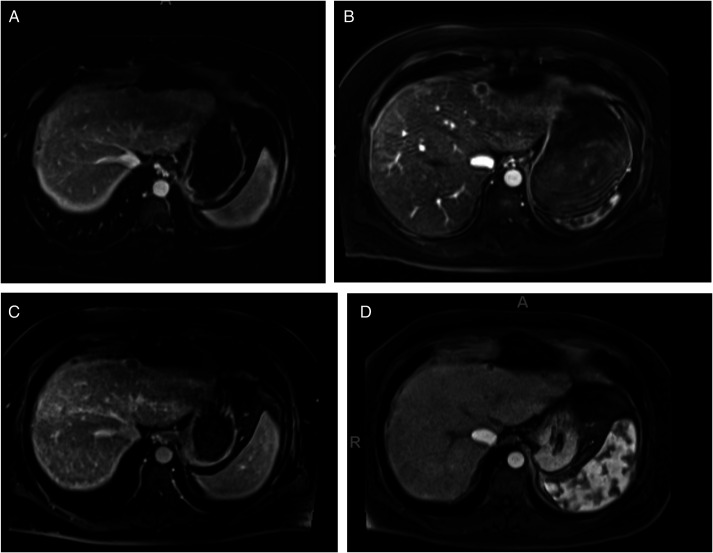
Imaging findings in patient 1: (A) gadolinium-enhanced magnetic resonance imaging (MRI) showing a new 1.5 × 1.3 cm LI-RADS 4 lesion in segment 2/4A. (B) Surveillance MRI 3 months later revealing an increase in size to 1.7 × 1.4 cm with arterial phase hyperenhancement and contrast washout on the venous phase (not shown) compatible with hepatocellular carcinoma (HCC) (LI-RADS 5). (C) A surveillance MRI performed 9 months after diagnosis of the liver lesion showed stable size and loss of arterial phase enhancement. (D) MRI 15 months after initial diagnosis of the liver lesion showing a reduction in size to 0.9 × 0.9 cm.
